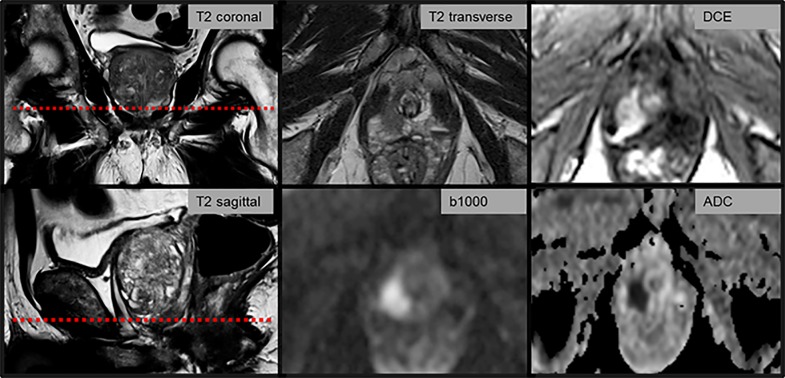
Example of PI-RADS scoring with version 1 and 2 in a patient with prostate cancer. mpMRI of an 82-year-old man with elevated PSA of 8.2 μg/l. The dominant lesion is located in the apex of the prostate in the pz and measures 12 x 18 x 17 mm. According to PI-RADS v2, DWI is the leading sequence. As the lesion has high signal intensity on b1000 with corresponding strong ADC reduction of 0.5 10^−3^ mm^2^/s as well as a diameter >15 mm the lesion was scored PI-RADS 5, highly likely to be malignant. No other sequence is required for scoring according to PI-RADS v2. With PI-RADS v1 a score for each sequence needs to be determined. For this patient the following scores were assigned: T2 TSE PI-RADS 5, DWI PI-RADS 5, DCE PI-RADS 5. This results in in a PI-RADS sum of 15 and a global PI-RADS 5. In this patient the time need for PI-RADS scoring with v2 after inspection of all images was 9 seconds, while it was 59 seconds with v1. MRI-guided in-bore biopsy revealed Gleason 3+4 = 7 tumor.
